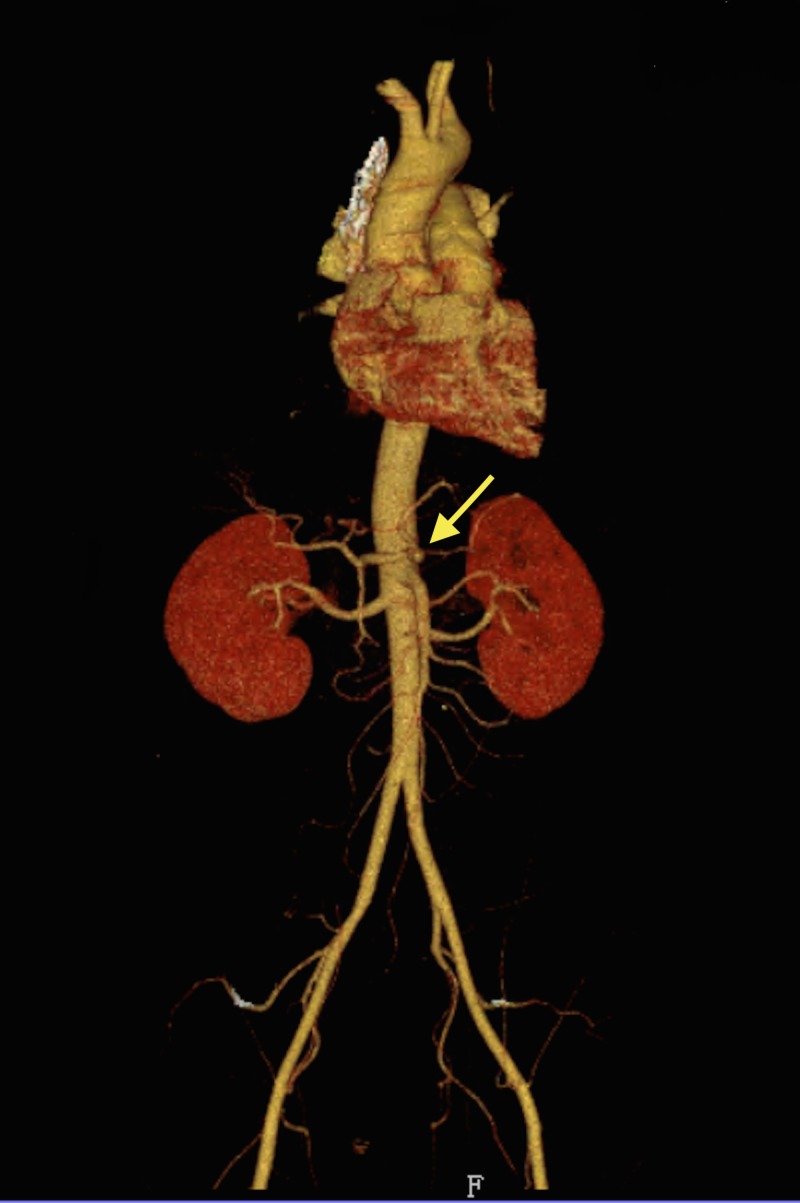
Computer tomographic (CT) three-dimensional reconstruction showing celiac artery dissection with proximal hepatic stenosis (arrow)
